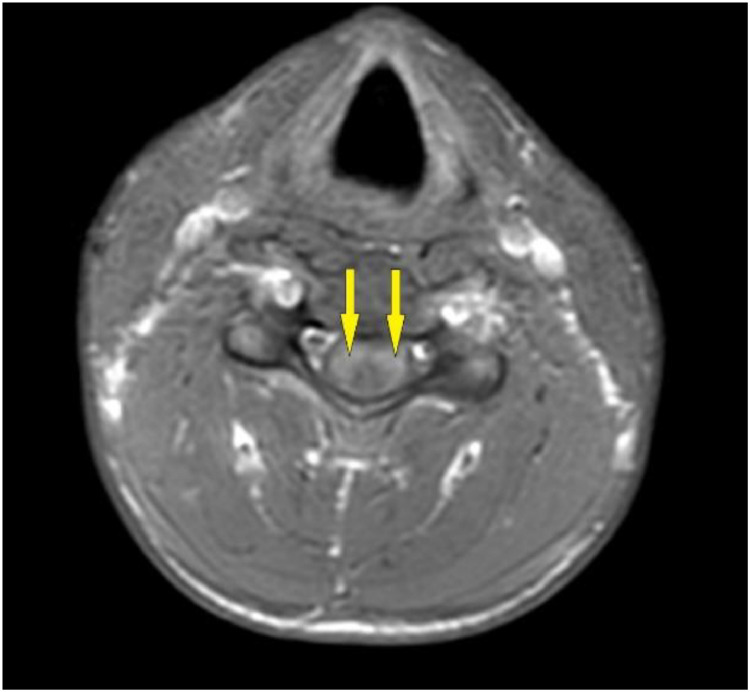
Axial MRI cervical spine T1 post contrast FAT SAT image showing enhanced peripherally placed lesion in upper cervical region.
